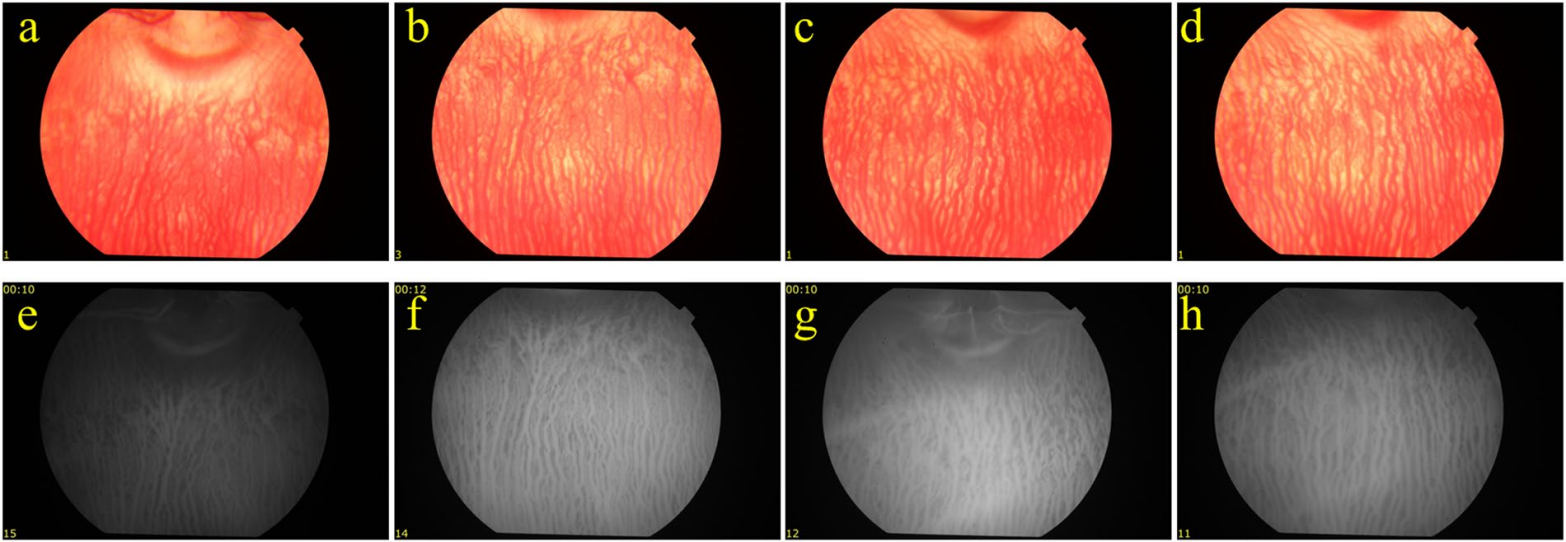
Fundus photographs and ICGA for the control cases where either only laser or only ultrasound was applied to the choroid layer. (a,b) Color fundus photos taken from a rabbit eye before and 4 weeks after the treatment using 75 mJ/cm^2^ laser pulses (no ultrasound burst applied). (c) and (d) Color fundus photos taken from a rabbit eye before and 4 weeks after the treatment using 0.5 MPa ultrasound bursts (no laser pulse applied). (e,f) ICGA results correspondent to the photos in (a,b) respectively. (g,h) ICGA results correspondent to the photos in (c,d) respectively.
